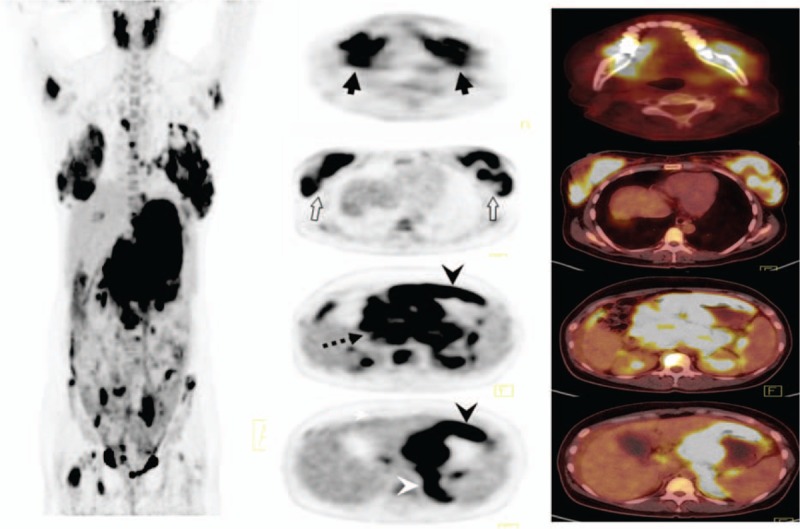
PET/CT images. PET/CT scan showed multiple hypermetabolic lesions in both mandible (black arrows), both breasts (white arrows), stomach (black arrowhead), pancreas (dotted arrow), and left adrenal (white arrowhead). PET/CT = positron emission tomography/computed tomography.
